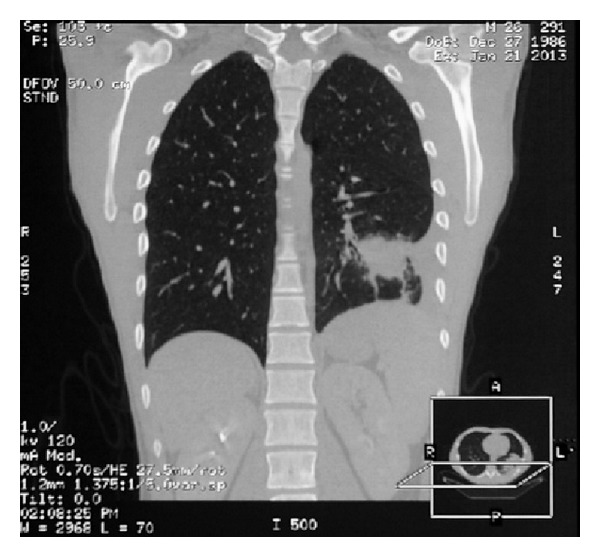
Tumor shadow was revealed in left lower lobe of the lung on chest CT scan.
